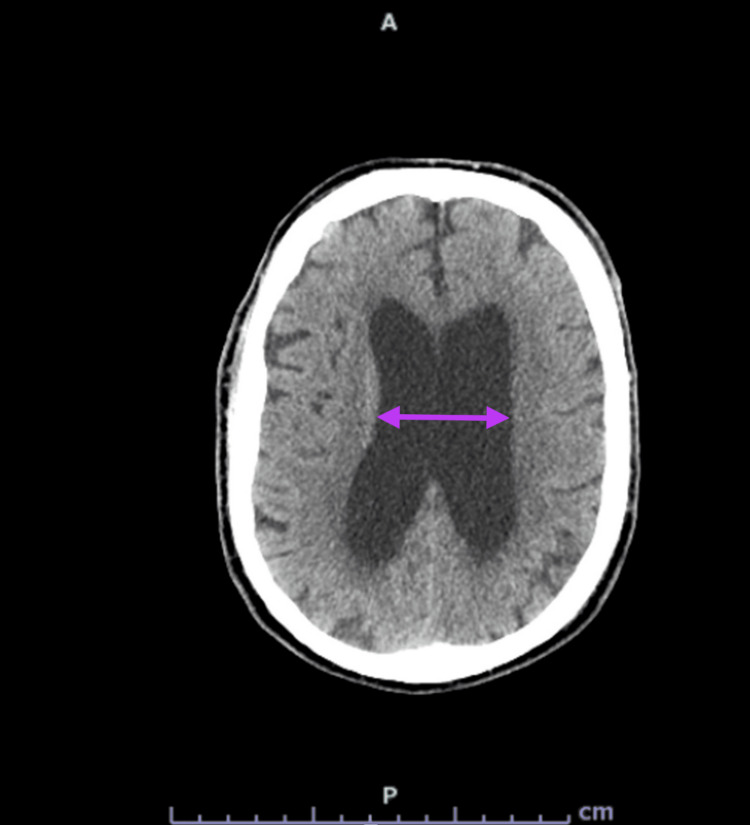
Non-contrast CT of the brain demonstrating ventriculomegaly. The arrows depict ventricular enlargement.
